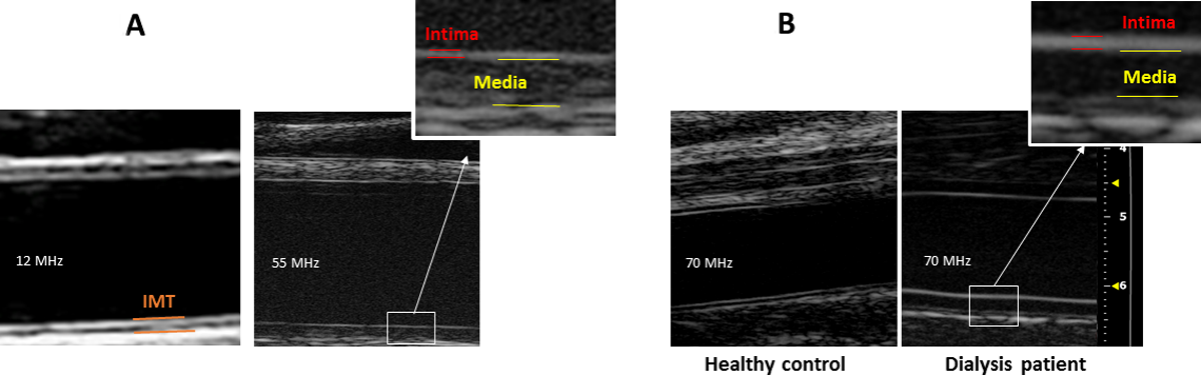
Ultra-high-frequency ultrasound images of carotid and dorsal pedal arteries, and comparison with images obtained by conventional ultrasound. Fig 1A—Carotid artery scanned with 12 MHz (left) and 55 MHz (right) ultrasound. Outtake shows magnification of intima-media complex from 55 MHz image with outline of intima and media thickness. Fig 1B—Dorsal pedal artery of control child (left) and child on hemodialysis (right) scanned with 70 MHz probe).
